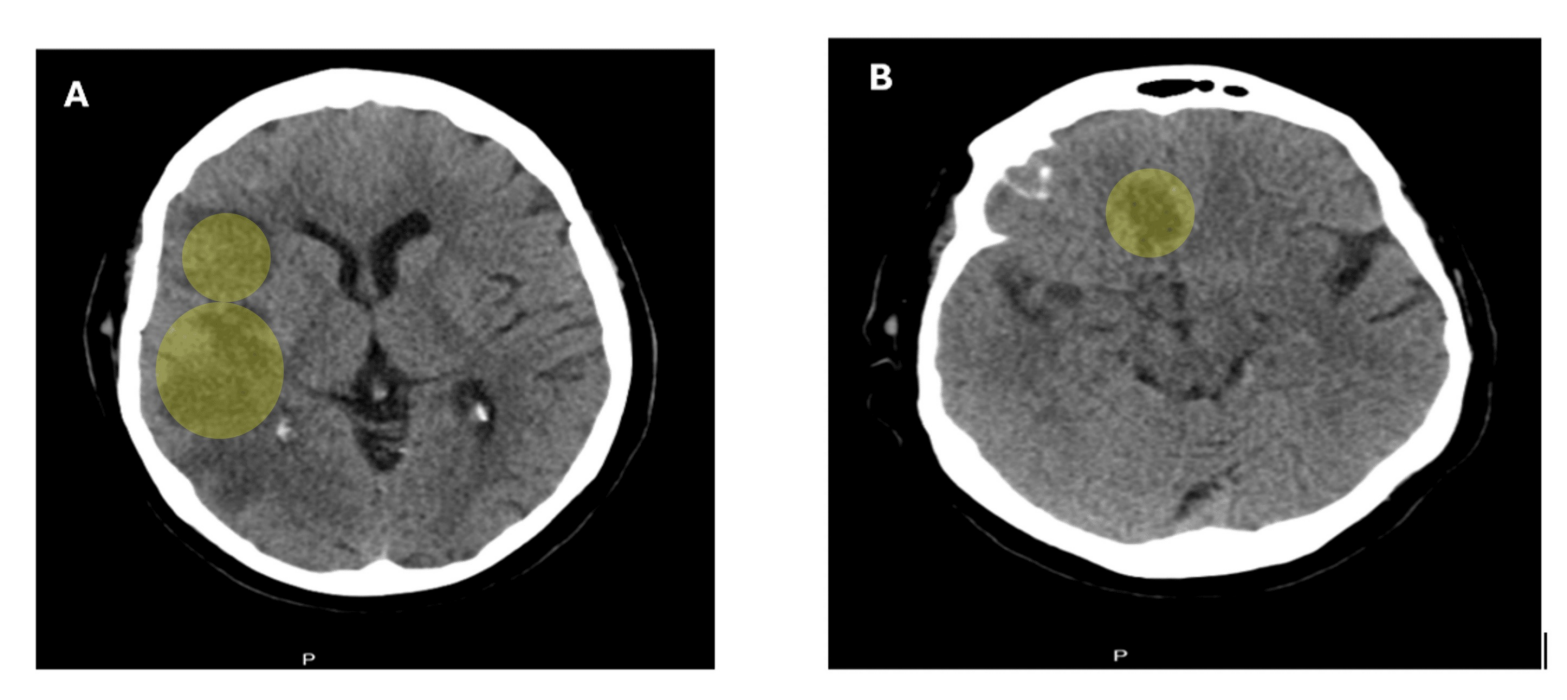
(A) Hypodensities in the right MCA territory involving the frontal, temporal, and parietal lobes on non-contrast axial CT of the brain (yellow highlight). (B) Hypodensity in the left frontal parafalcine region on non-contrast axial CT of the brain (yellow highlight). MCA, middle cerebral artery; CT, computed tomography
